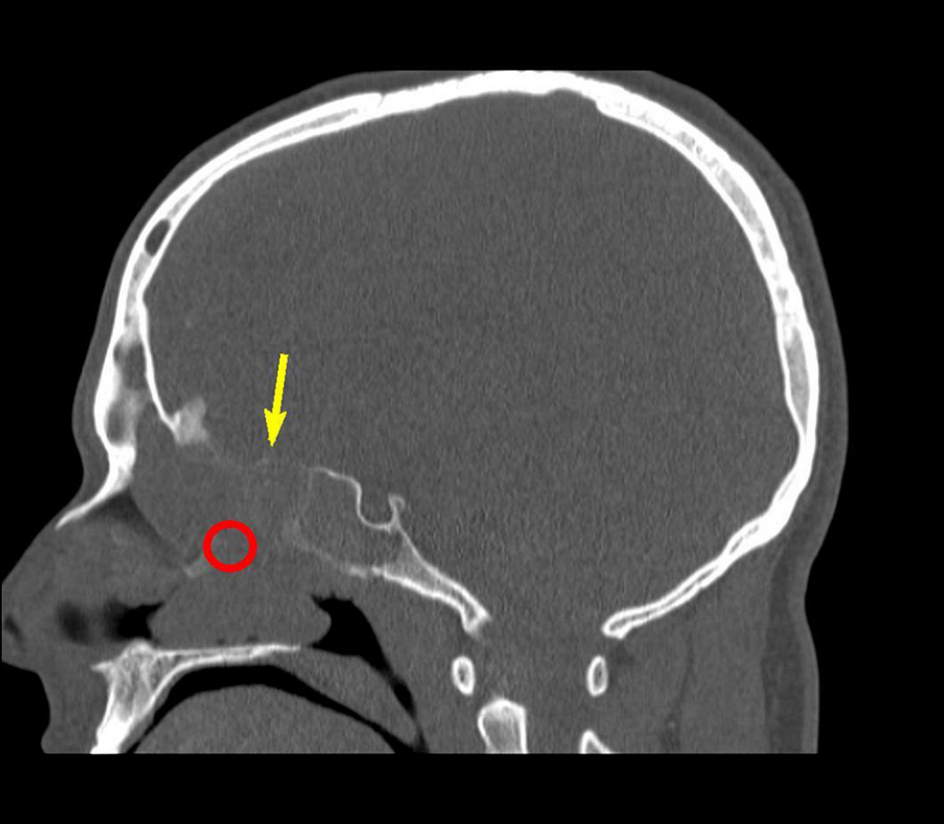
Sagittal non-enhanced CT imaging in bone window shows a soft tissue mass (red circle) and focal erosive bone changes of the orbital part of the left frontal bone (yellow arrow).
